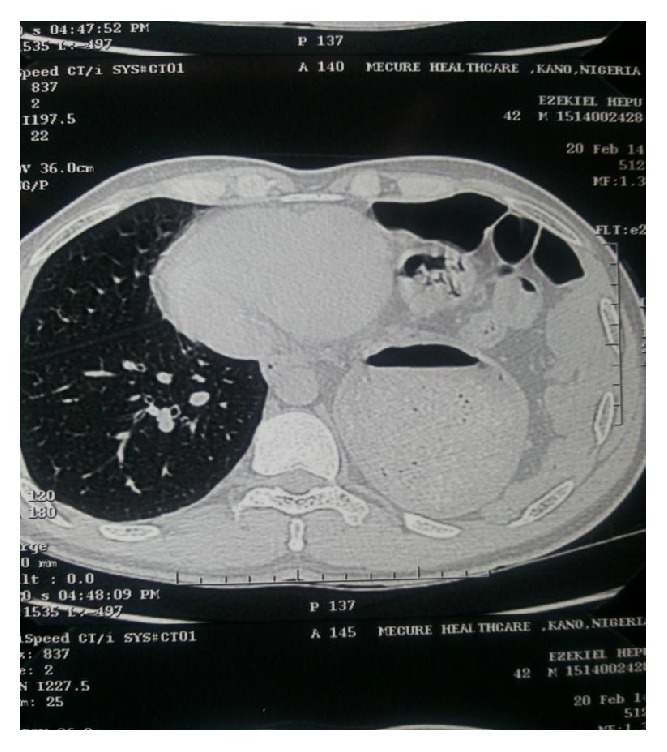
Computed tomography (CT) showing presence of stomach and bowel loops in the chest.
